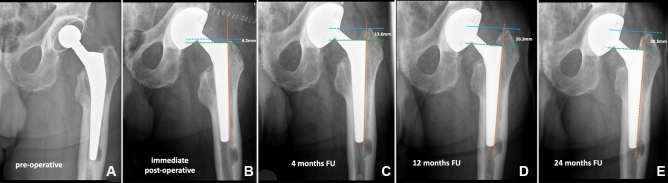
A–E show progressive subsidence of the revised stem due to undersizing (aseptic loosening). The stem size was 24, the biggest size for this specific model. A shows a pre-operative X-ray. The reason for the revision was aseptic loosening. B shows immediate post-operative X-ray control. Subsidence was 9.4 mm at 4 months of FU (C), 16.1 mm at 12 months of FU (D), and 16.3 mm at 24 months of FU (E). *FU* follow-up
